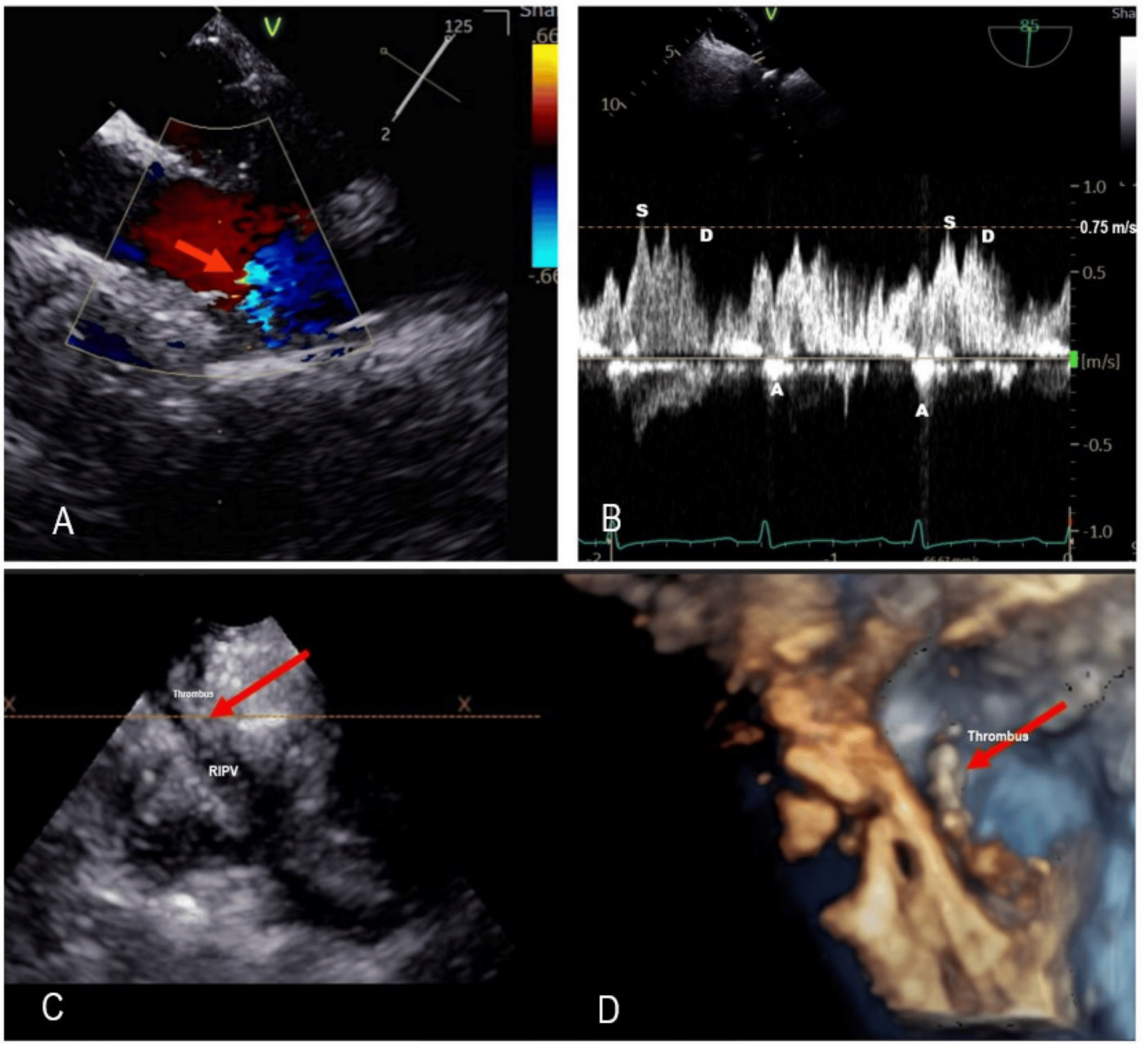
TEE 2D TEE of the RIPV performed at 45º-60º showed turbulent flow seen with CF-Doppler (4A) and PW-spectral Doppler has pulmonary vein S & D waves velocities at 0.75 m/s (4B); both findings are associated with pulmonary vein thrombosis and obstruction. 2C. 45º-60º shows the pulmonary vein had a small ostium caliber with an echogenic structure decreasing its lumen. The 3D TEE (2D) shows the echogenic structure is a thrombus. 2D TEE: two-dimensional transesophageal echocardiogram; 3D TEE: three-dimensional transesophageal echocardiogram; RIPV: right inferior pulmonary vein; PW-Doppler: pulse wave Doppler; CF-Doppler: color flow Doppler; S wave Velocity: pulmonary systolic vein flow; D wave velocity: pulmonary diastolic vein flow
